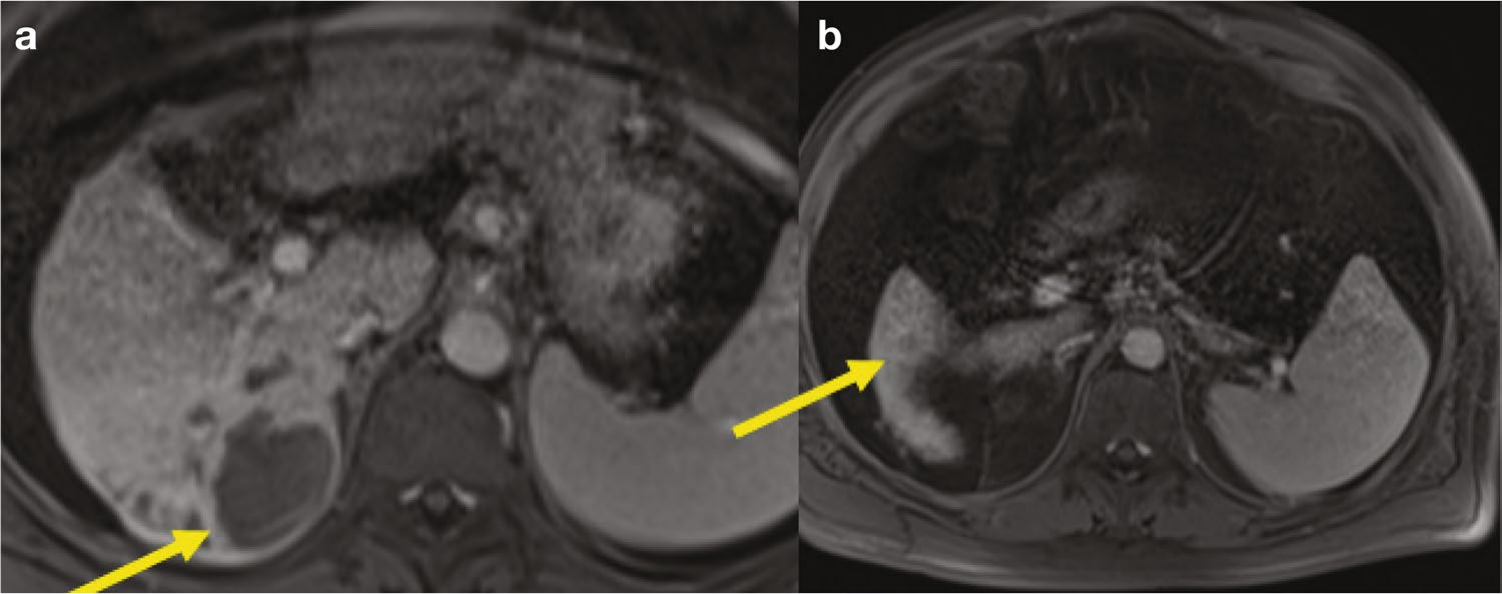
Same patient as in Fig. 3. Gadoxetic acid-enhanced T1-weighted images post-TARE during the PVP at two different levels demonstrate completely necrotic lesion in segment 7 (a), and persistently enhancing lesion in segment 6 (arrows) (b). *Abbreviations: PVP,* portal venous phase*; TARE,* transarterial radioembolization
